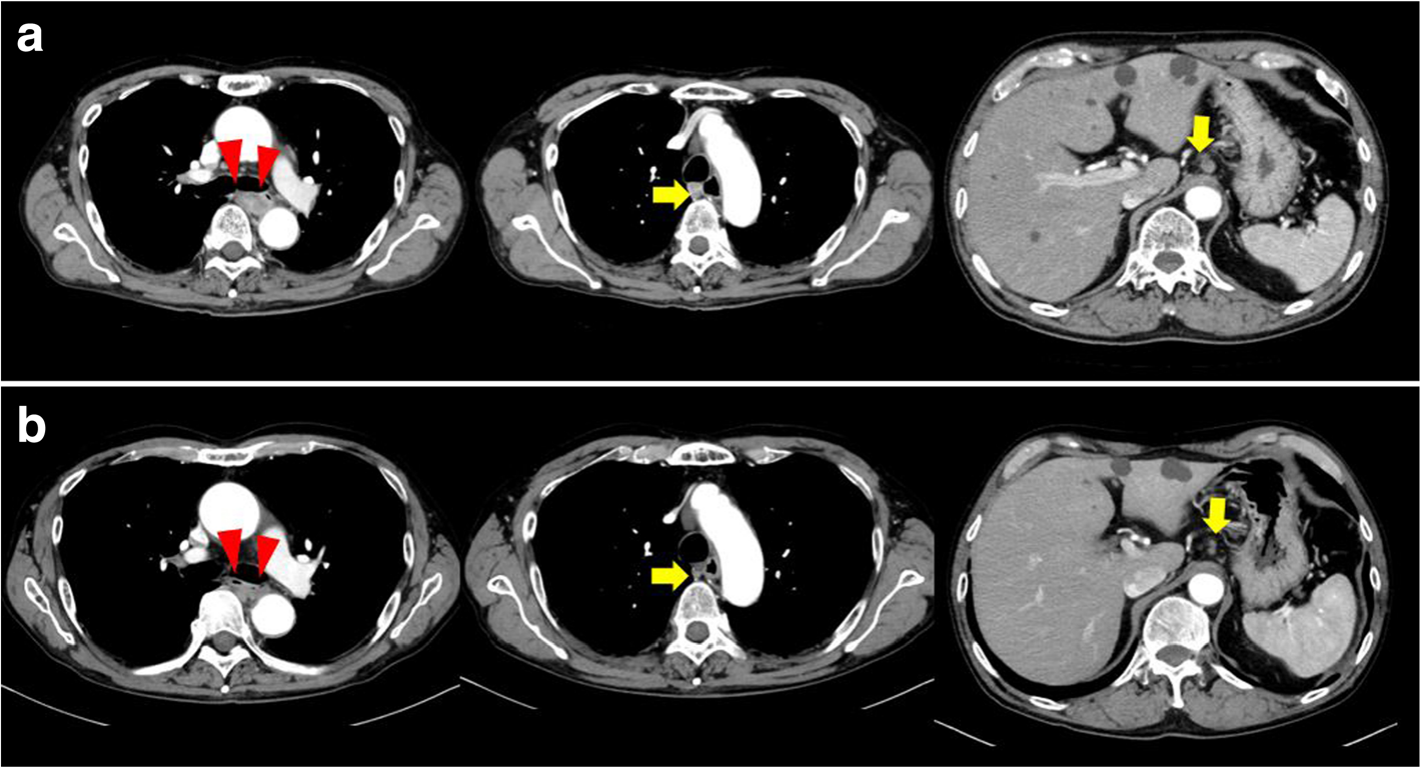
Computed tomography findings. a Before preoperative chemotherapy, a tumor was observed in the middle of the esophagus (red arrows) with swollen lymph nodes in the mediastinum and around the cardia (yellow arrows), but there was no distant metastasis. b After preoperative chemotherapy, the tumor (red arrows) and swollen lymph nodes in the mediastinum and around the cardia (yellow arrows) were reduced
